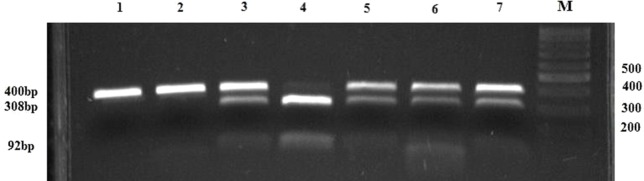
PCR-RFLP restriction products of the *GSTA1* gene. Lanes 1 and 2 represent PCR products of *GSTA1CC genotype (400 bp bands); lanes 3, 5, 6, and 7 represent PCR-RFLP restriction products of *GSTA1CT genotype (400 bp, 308 bp, 92 bp bands); Lane 4 comprises RFLP-PCR restriction products of *GSTA1TT genotype (308 bp, 92 bp bands); M, DNA marker; N, negative control without a DNA content.
